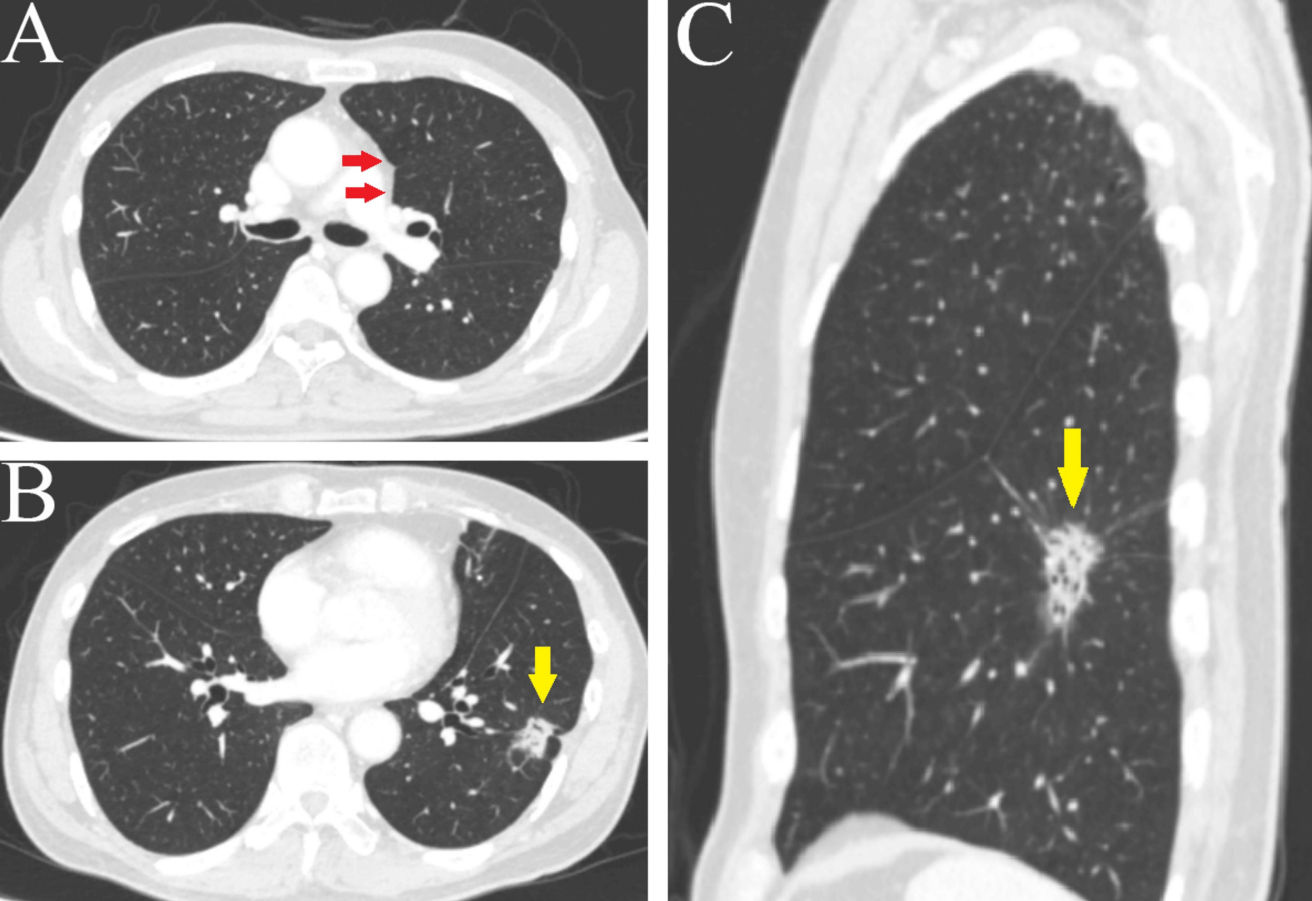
Contrast-enhanced CT findings (A) No emphysematous changes or inflammation were observed at the pneumatocele site (red arrow). (B) Horizontal view: a part-solid nodule measuring 1.6 × 1.4 cm, with a 1.5 cm solid component, was detected in S9/10 of the left lower lobe (yellow arrow). (C) Sagittal view: a part-solid nodule with a maximum diameter of 4.6 cm and a solid component measuring 4.1 cm was observed (yellow arrow).
